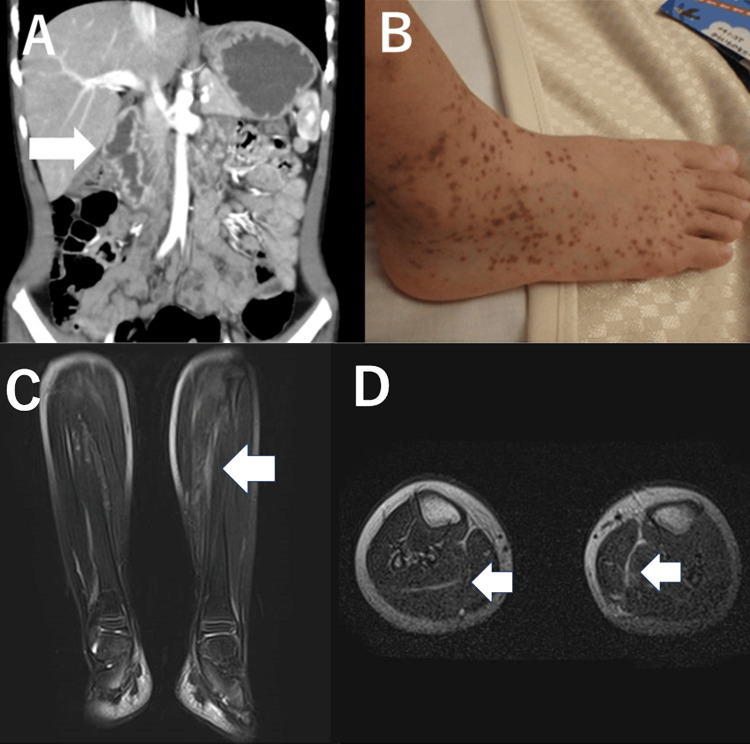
A. Abdominal contrast-enhanced computed tomography showing contrast enhancement of the duodenum with fluid retention. B. Palpable purpura on the leg. C and D. Fat-suppressed (chemical shift selective saturation (CHESS)) magnetic resonance imaging of the lower thigh shows high-signal areas around the soleus muscle.
